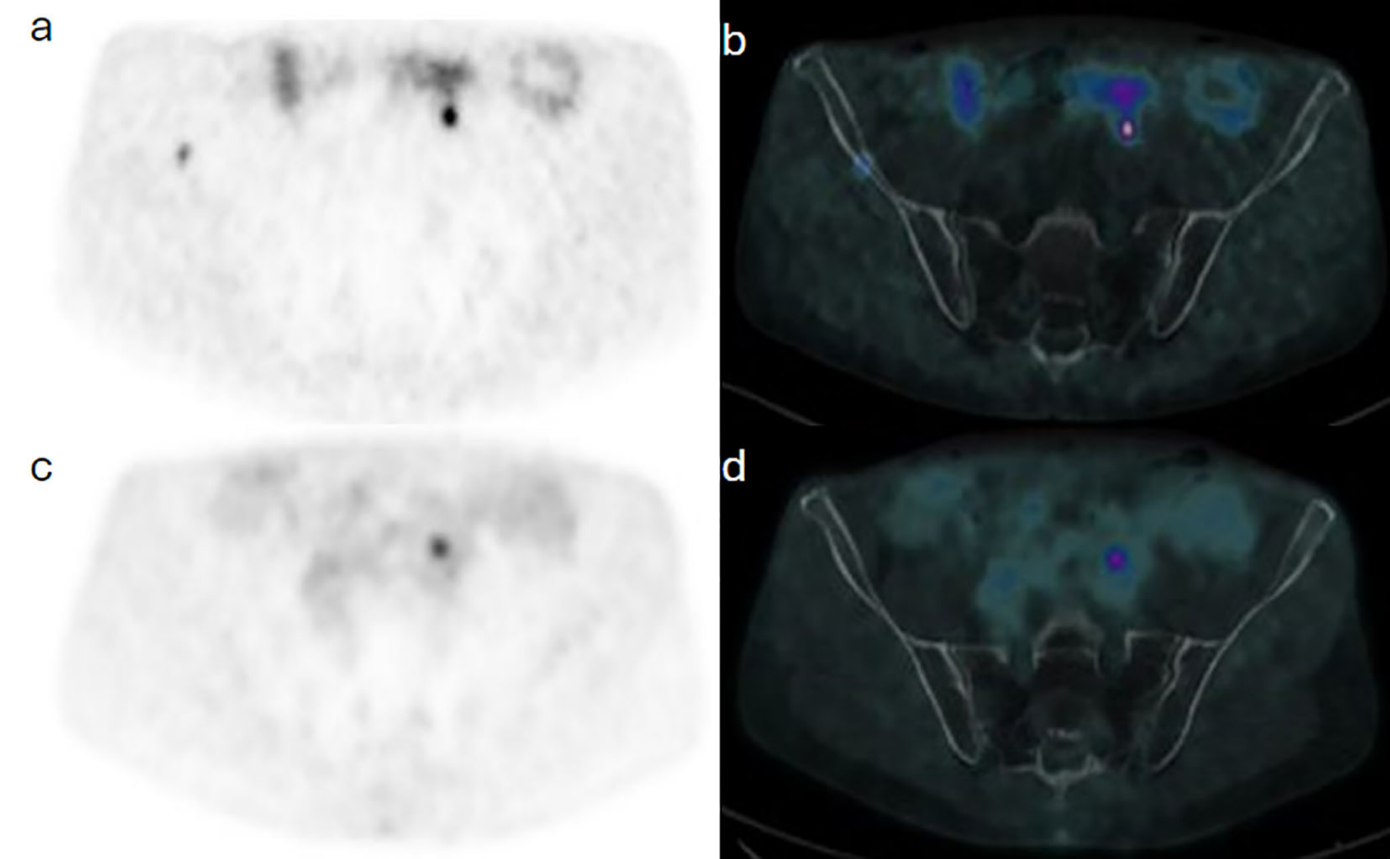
Case 3. Trans-axial view images at the pelvic inlet level show a focal of Ga68-DOTATATE on PET (a) and PET/CT (b) components of the study, with no correspondent F18-FDG activity on PET (c) and PET/CT (d) images of subsequent images.
